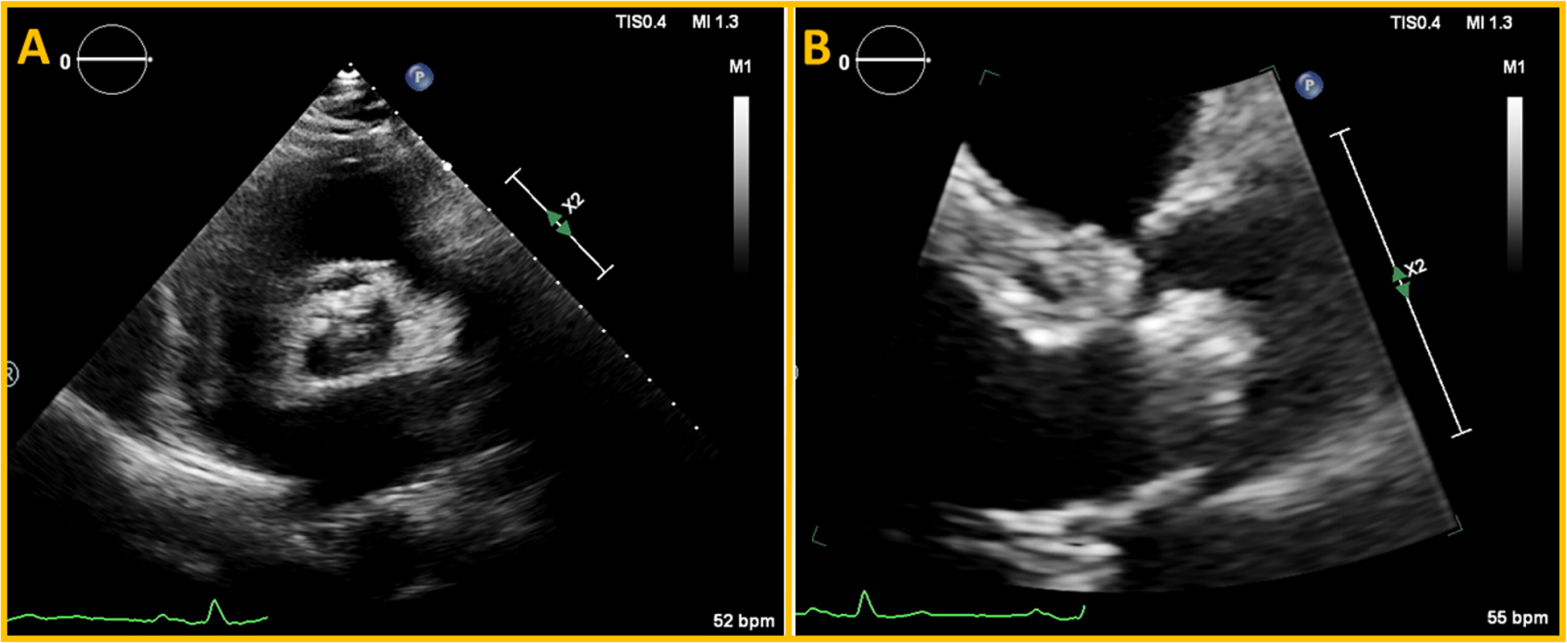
Aortic valve calcification in parasternal short axis (a) and long axis views (b)
